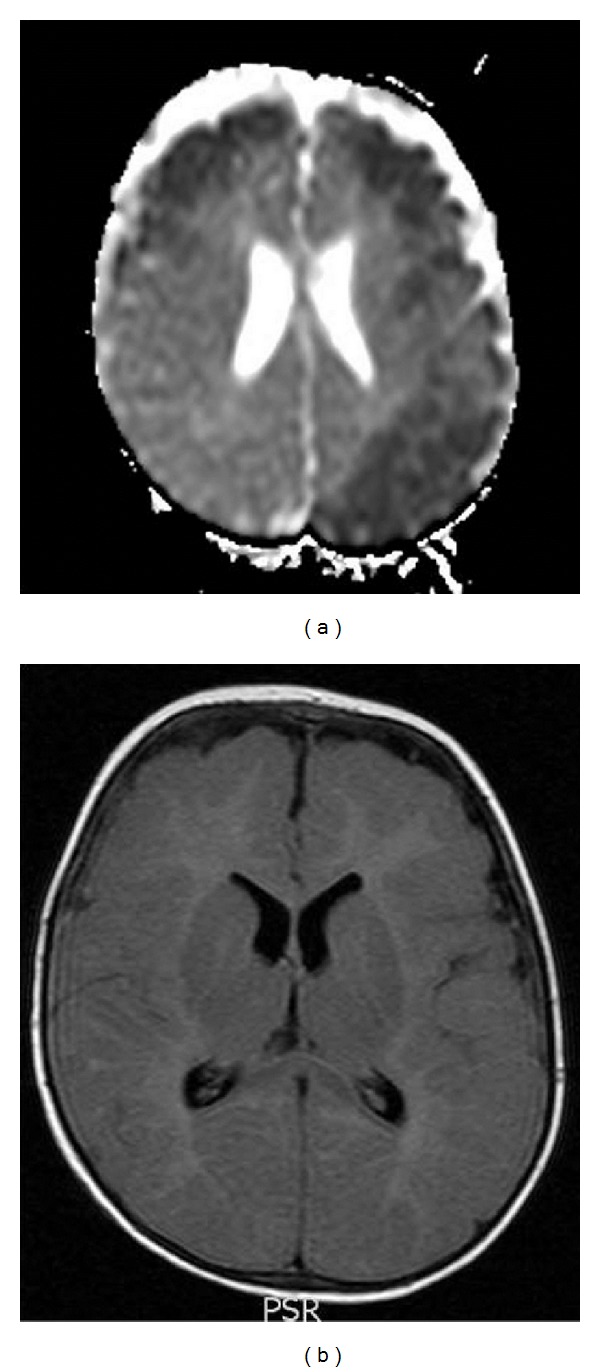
(a), (b) Brain magnetic resonance imaging one day after admission. (a) Axial apparent diffusion coefficient (ADC) map shows large areas of cortical and subcortical restricted diffusion in the frontal areas bilaterally, left parietal and left temporal regions, suggestive of encephalitis. (b) Fluid attenuated inversion recovery (FLAIR) sequence showing normal brain tissue.
